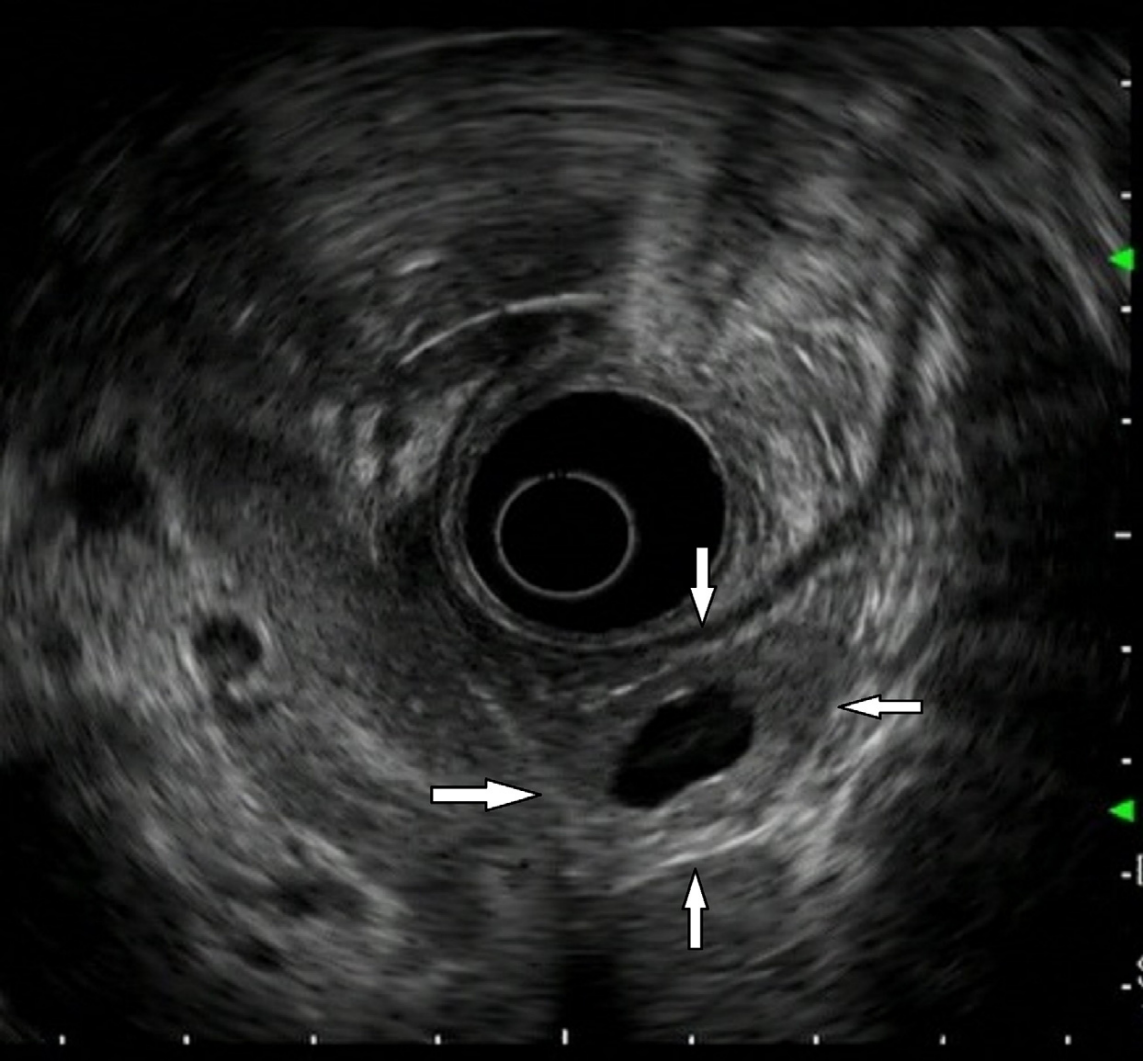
Endoscopic ultrasonography revealed a 25‐mm cystic lesion (white arrows) in the pancreatic tail.
